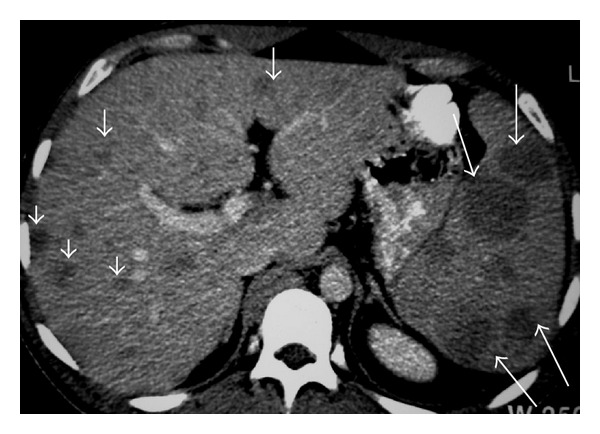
NHL. Axial contrast-enhanced CT image shows low-attenuation nodules in the liver (short arrows) and spleen (long arrows).
